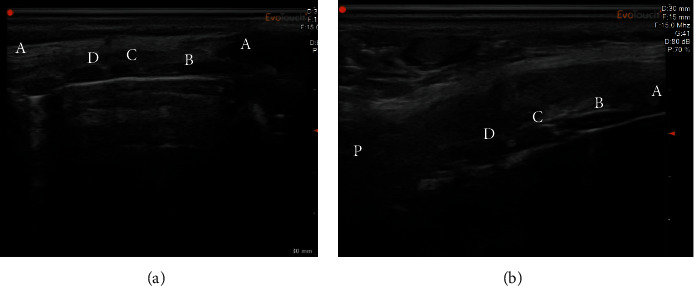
(a) Longitudinal ultrasound image of the neck: (A) cricoid cartilage, (B) 1st tracheal ring, (C) 2nd tracheal ring, and (D) 3rd tracheal ring. (A) Anterior side. (b) Longitudinal ultrasound image of the neck: (A) cricoid cartilage, (B) 1^st^ tracheal ring, (C) 2^nd^ tracheal ring, and (D) (3^rd^) tracheal ring. (P) Posterior side.
